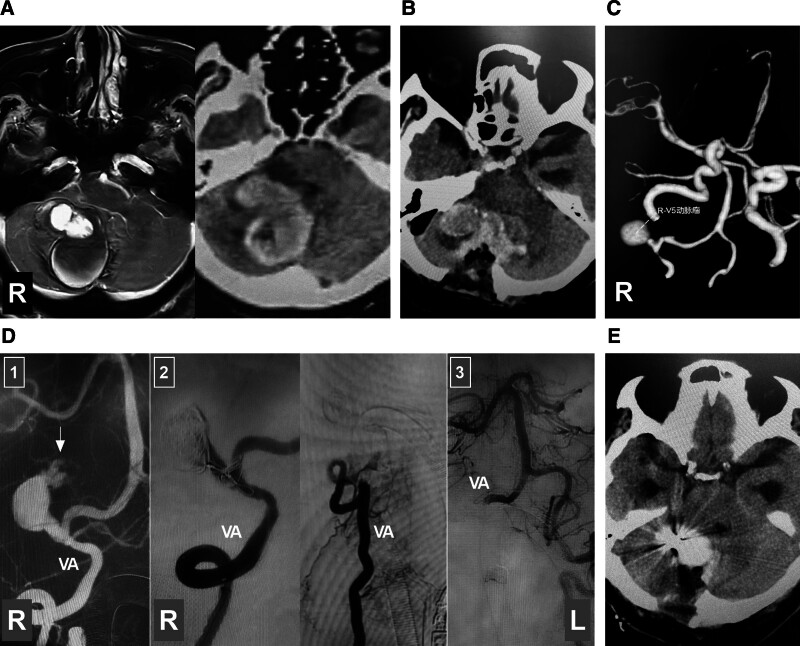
Combined EVT and aneurysmectomy in treating a giant intracranial VA aneurysm. (A) Enhanced magnetic resonance (left panel) and CT (right panel) images showing a lesion with a mass effect in the posterior fossa. (B) CT image showing the area of interest after exploratory craniotomy. (C) CT angiography image confirming that the lesion is an aneurysm. (D) Panel 1: Roadmap image showing the rupture point (arrow) of a giant VA aneurysm; panel 2: DSA image of the right VA showing occlusion of the aneurysm and parent VA with coils; panel 3: DSA image of the left VA showing that the vessel supplies the right VA distal to the aneurysm and basilar artery. (D) Postoperative CT showing the results of aneurysmectomy and resolution of the mass effect. CT = computed tomography, DSA = digital subtraction angiography, L = left, R = right, VA = vertebral artery.
